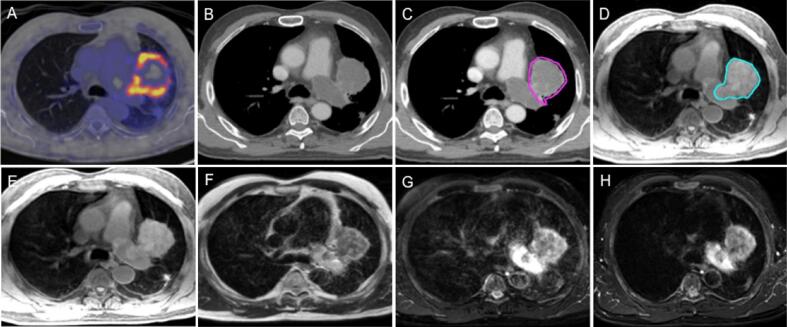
Intravascular invasion; (A – H): Same transverse slice showing invasion into the pulmonary artery and intravascular thrombus, (A) PET-CT scan, (B) CT scan (L: 46, W:600 HU) (C) CT scan with CT consensus contour (pink), (D) fat-suppressed T_1_w Radial GRE with MR consensus contour (light blue), (E) T_1_w Radial GRE, (F) T_2_ TSE non-fat-sat, (G) T_2_w TSE fat-sat, (H) T_2_ TSE Dixon Water. (For interpretation of the references to colour in this figure legend, the reader is referred to the web version of this article.)
